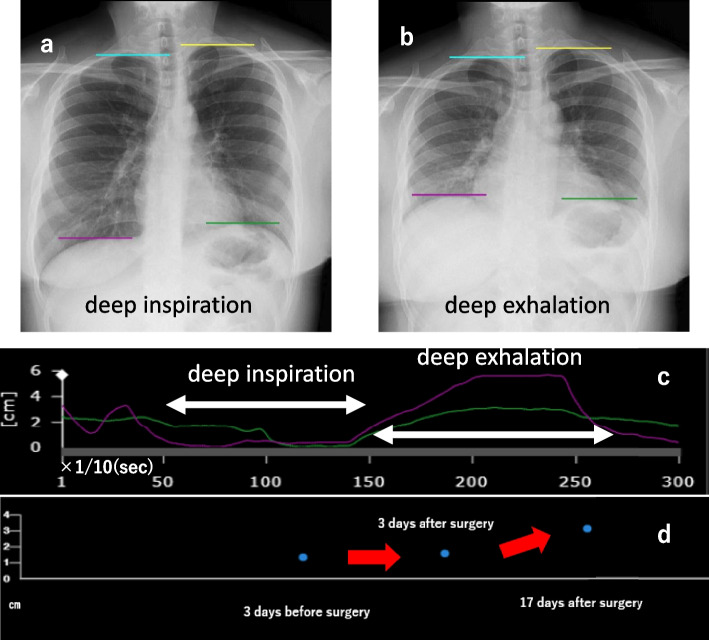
Details of dynamic digital radiography image. a Dynamic digital radiography image during deep inspiration 17 days after surgery. b Dynamic digital radiography image during deep exhalation 17 days after surgery. c Displacement of the left and right diaphragm over time during a single breath. d The patient showed improvement in left diaphragmatic movement over time
